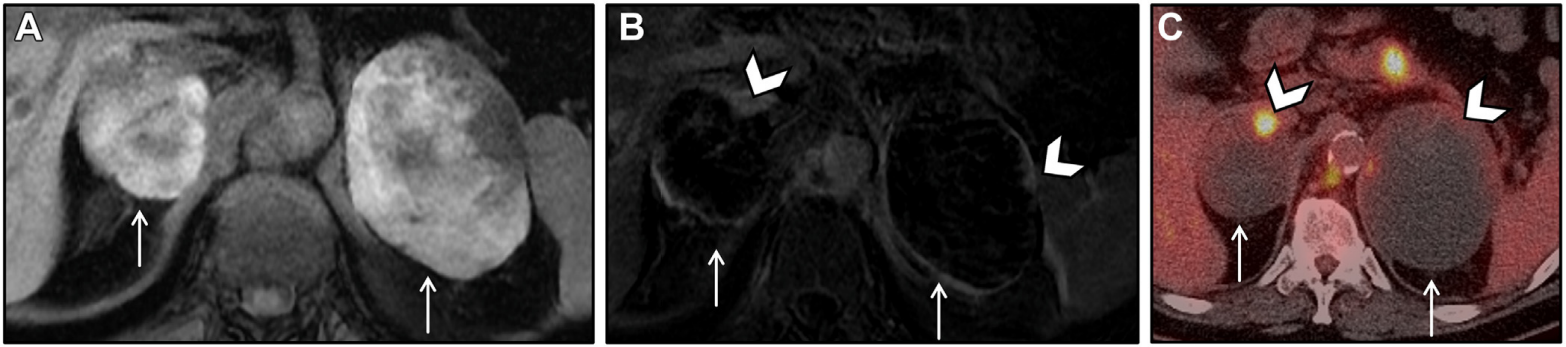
Bilateral adrenal hemorrhage due to malignancy. (*A*) Axial T1-weighted MR image showing bilateral adrenal lesions (arrows) with intrinsic T1 hyperintensity consistent with hemorrhagic lesions. (*B*) Axial subtracted MRI post contrast image showing only small areas of peripheral enhancement (arrowhead) with much of the lesion without enhancement. Findings consistent with bilateral metastatic disease with hemorrhage. (*C*) Axial fused FDG PET/CT image in the same patient showing the bilateral lesions with minimal focal peripheral increased FDG uptake correlating to the areas of enhancement seen on the MRI, related to viable tumor with adjacent hemorrhage. *CT* = computed tomography; *FDG PET* = fluorodeoxyglucose positron emission tomography; *MRI* = magnetic resonance imaging.
